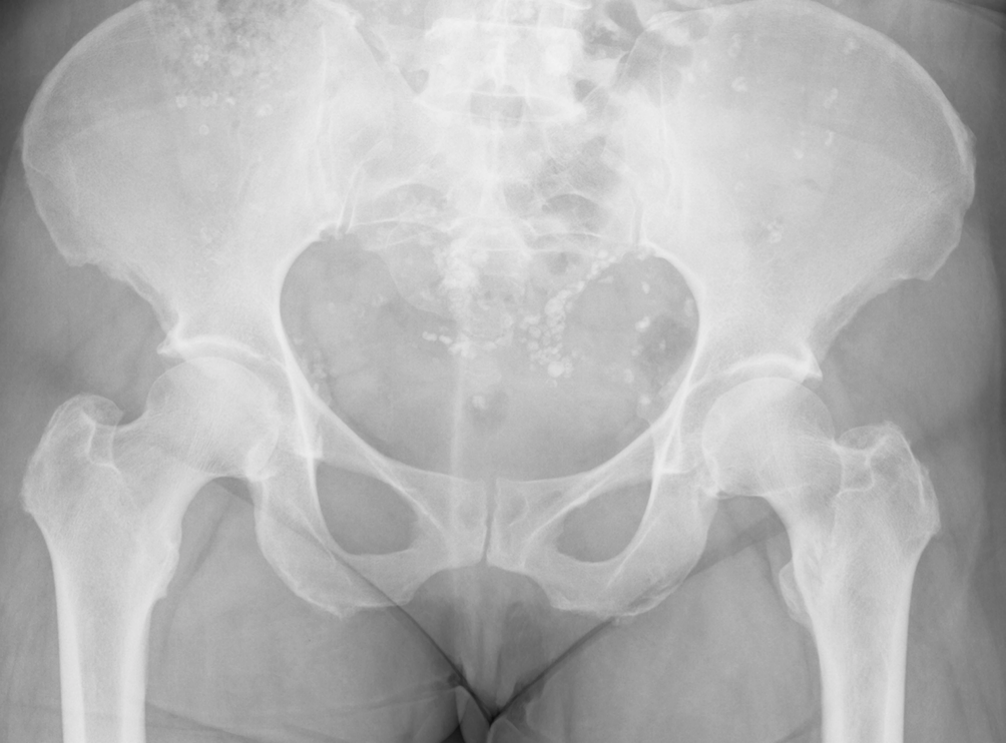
Pelvic radiograph following a fall. Multiple calcific densities throughout
